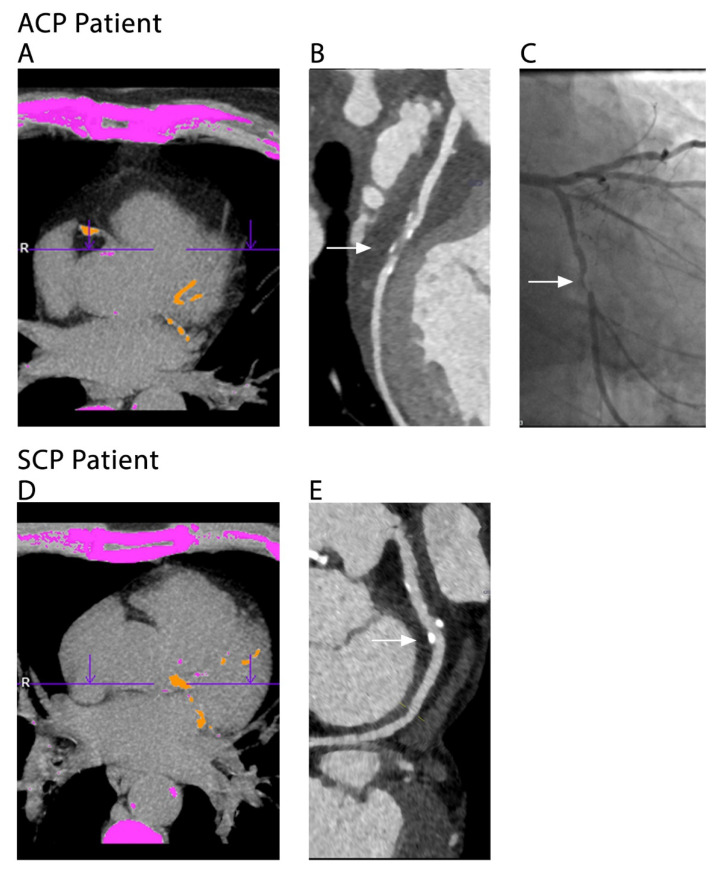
CT scan examples of an ACP and a SCP patient. (A–C) 67-year-old male ACP patient with a history of hypertension, hyperlipidemia and smoking. (A) CACS scan of 461 au. (B) CCTA shows a severe calcified lesion in the circumflex artery (CX) (arrow). (C) Subsequent ICA demonstrates the same severe lesion in the CX (arrow). (D,E) 65-year-old male SCP patient with a history of hyperlipidemia and smoking. (D) CACS scan of 418 au. (E) CCTA shows a non-obstructive calcified lesion in the CX. ICA was not performed since it was not indicated. ACP: acute chest pain, au: Agatston Units, CACS: coronary artery calcium score, CCTA: coronary CT angiography, CX: circumflex artery, ICA: invasive coronary angiography, SCP: stable chest pain.
